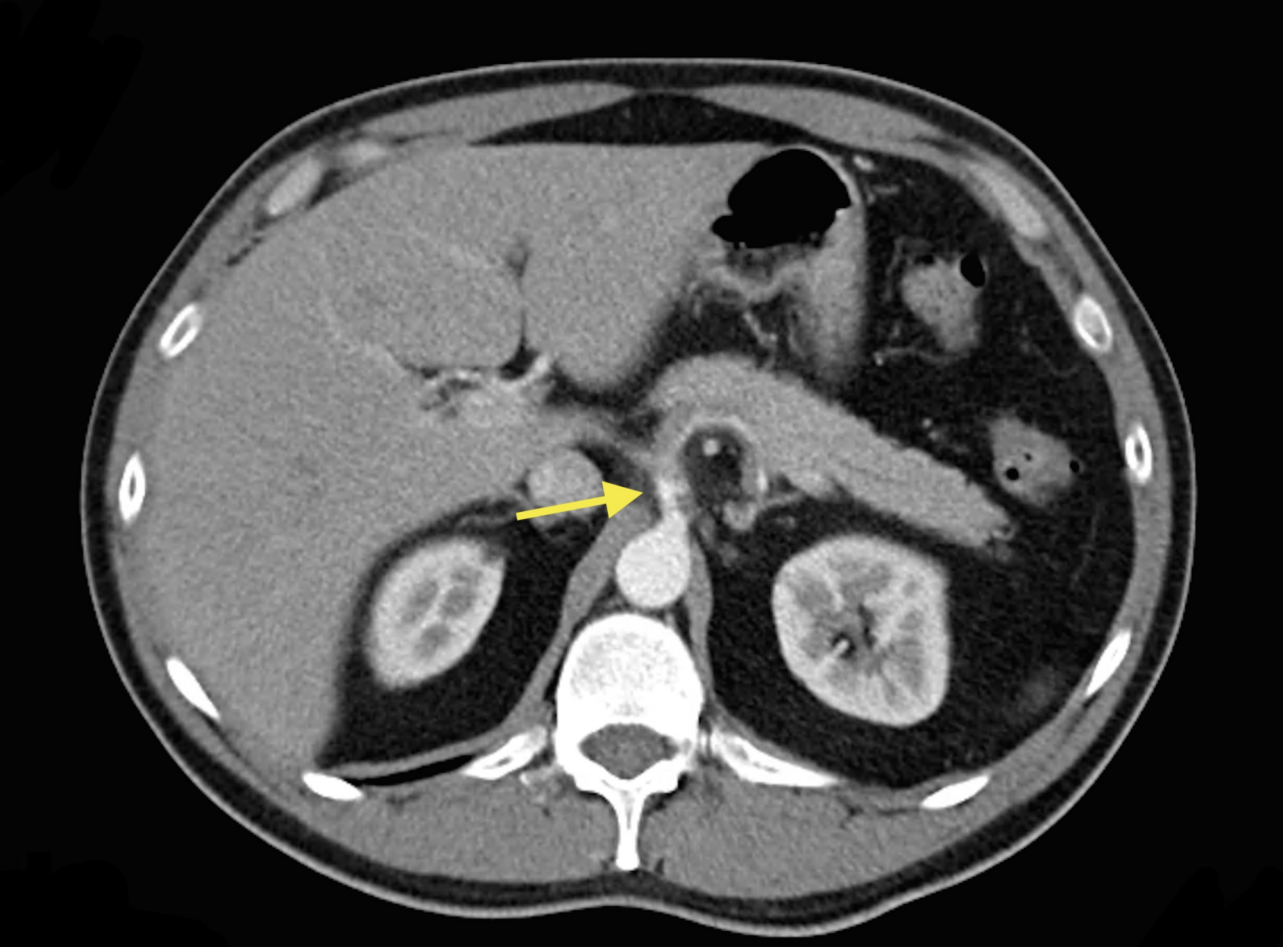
Computerized tomography (CT) of the abdomen and pelvis with contrast showed focal dissection of the celiac artery (arrow)
